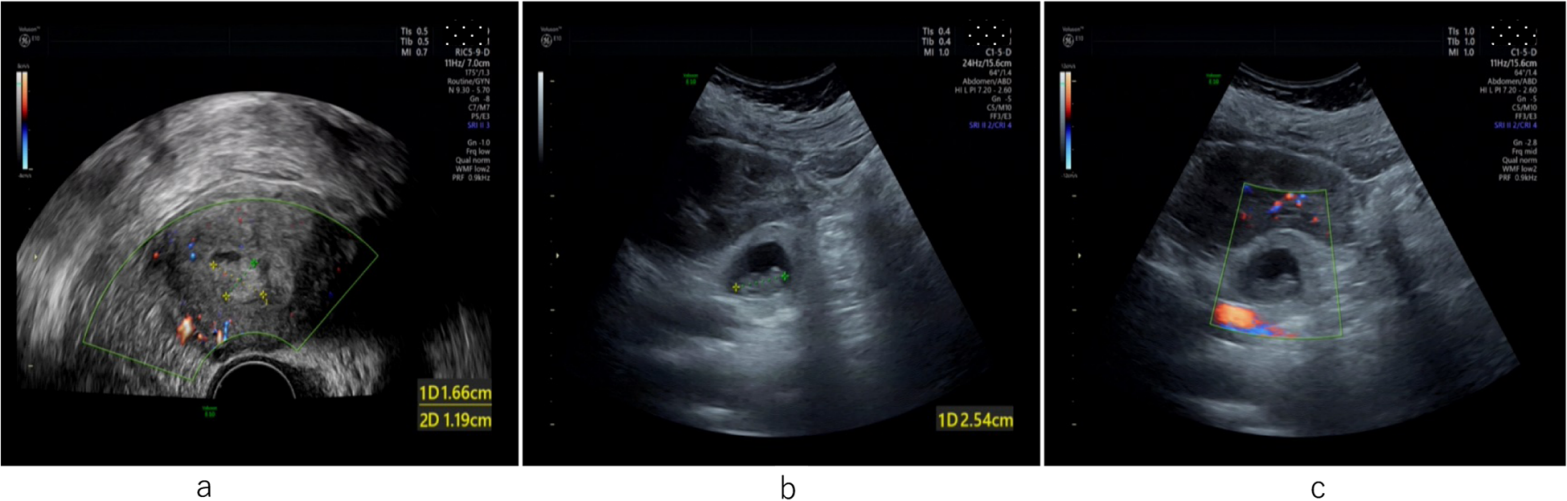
The ultrasonograms before the laparotomy . a transvaginal ultrasonogram of uterus after curettage; b: the feotal bud of retroperitoneal pregnancy by transabdominal ultrasonogram; c: transabdominal colour Doppler ultrasonogram of retroperitoneal pregnancy
